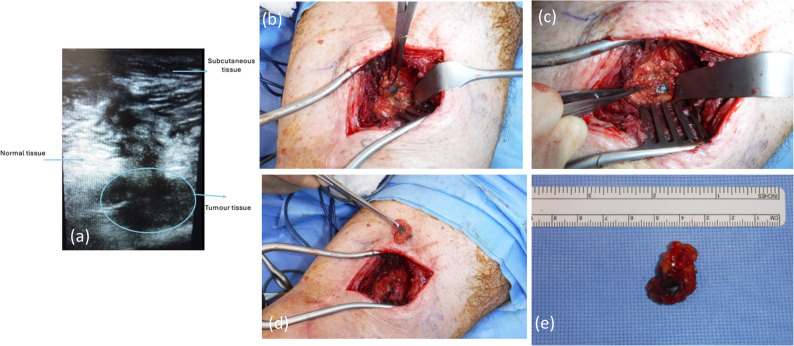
An example demonstrating the use of intraoperative ultrasound to guide tumour excision; (a) tumour identified on PET CT scan was intraoperatively localised with a portable ultrasound scan, (b) an initial excision at lesion site was made and the neurovascular bundle was retracted, (c) intraoperative ultrasound visualised tumour site and guided excision, (d) tumour excised (e) tumour measure around 1 × 1 cm
